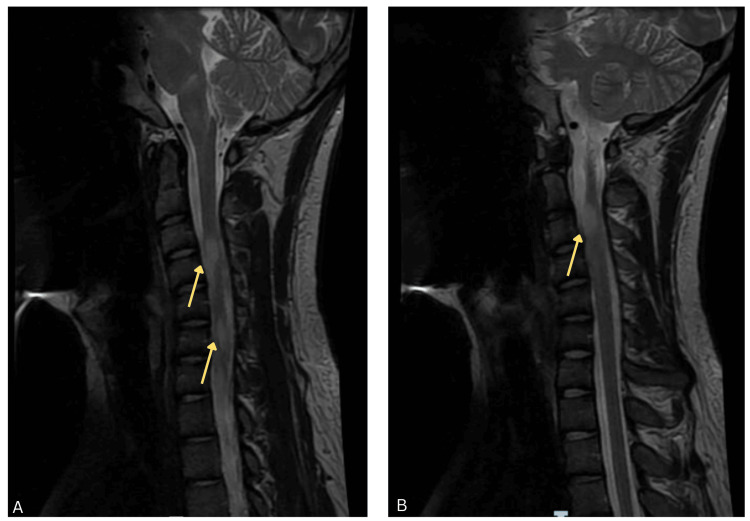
Cervical spine MRI of a 32-year-old male with multiple sclerosis (MS) A, B: sagittal T2-weighted sequences showing multifocal, hyperintense intramedullary lesions within the cervical spinal cord (yellow arrows). These characteristic short-segment plaques are classic manifestations of spinal cord demyelination in MS.
